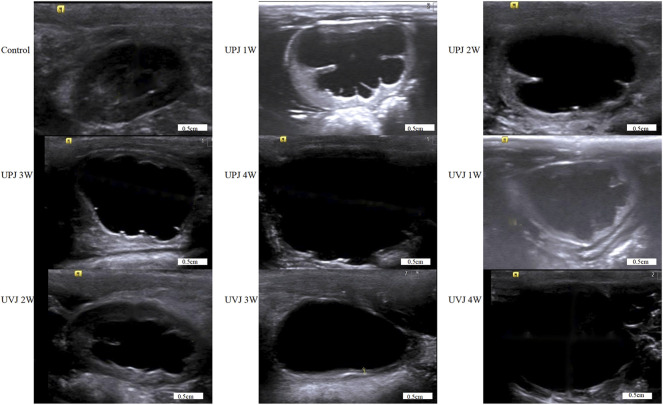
Images of renal pelvis in each group were obtained by B-ultrasound.
